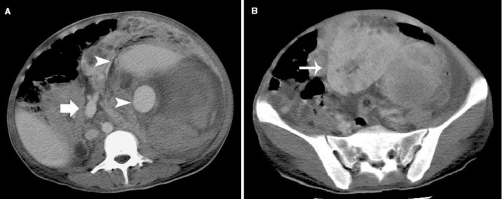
CT scans on admission. Abdominal CT demonstrated a fairly large-sized AML in the left retroperitoneum and containing a huge aneurysm-like hemorrhage (arrow heads). The left renal artery was dilated to nearly the size of the aorta (thick arrow). (B) A part of the normal renal parenchyma was identified (thin arrow).
